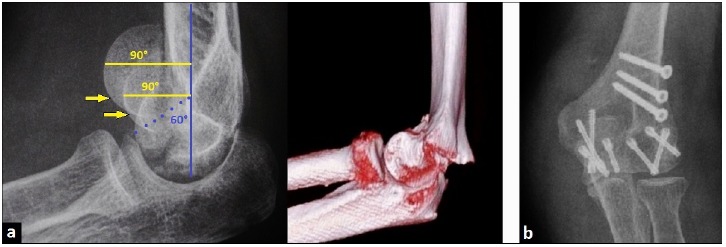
(Case Presentation): (a) Lateral radiograph and 3D CT scan demonstrating CSF type IV with anterior displacement of trochlea as well as capitellum of 30° (lines, points), note the pathognomonic "double arc sign" (arrows). (b) PA radiograph after primary ORIF showing correct alignment of the distal part of humerus without step-off in both articular surfaces.
